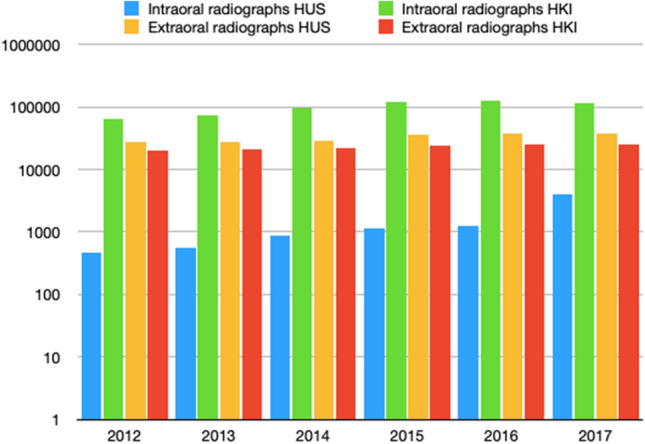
Amount of dental radiographs taken during 2012–2017: the rapid, almost threefold, increase in the number of intraoral radiographs from 2016 to 2017 (HUS) is due to the organizational change in which the dental students’ clinical practice was transferred from HKI to HUS
